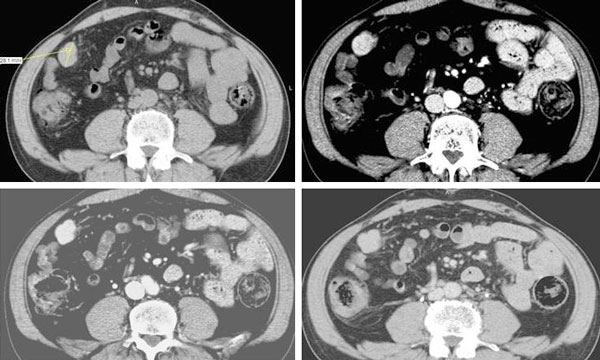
Abdominal CT scan of a splenic nodule before and after contrast medium injection. This 28 mm peritoneal nodule located in the right paracolic space showed slight hypodensity during the pre-contrast phase, (a) inhomogeneous contrast-enhancement during the arterial phase (b), slightly iso-hypodenity during the portal (c) and equilibrium phase (d).
